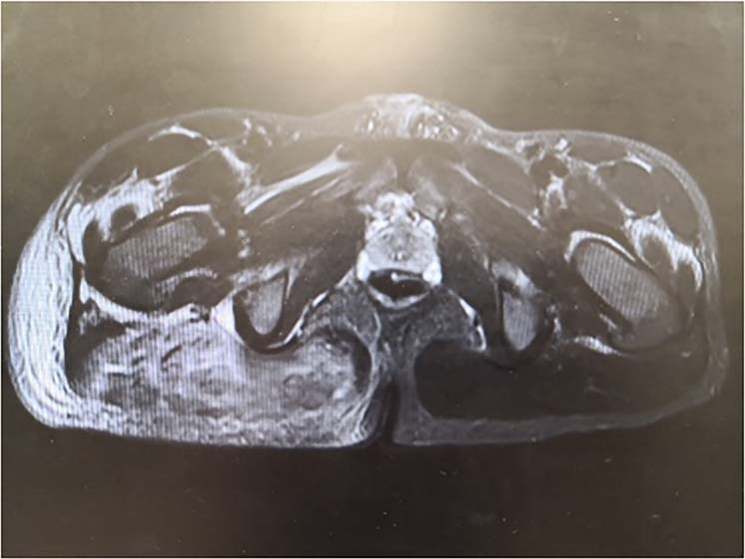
MRI pelvis demonstrating extensive inflammation of the right gluteus maximus with subcutaneous oedema.
